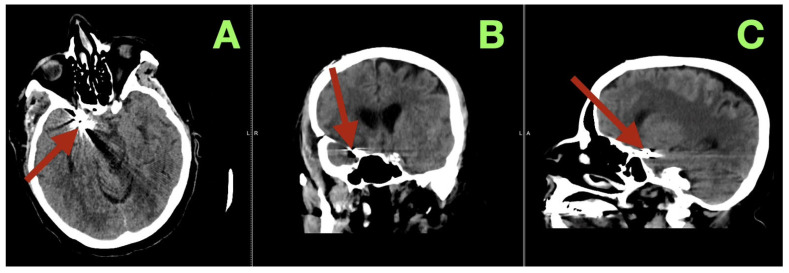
Postoperative day 5 non-contrast CT. (A) Axial section at the level of the skull base demonstrates the aneurysm clip at the right carotid–posterior communicating region (arrow), with expected localized metallic artifact and no evidence of recurrent subarachnoid hemorrhage, new intraparenchymal bleeding, or territorial hypodensity. The surrounding basal cisterns at this level remain discernible, without mass effect. (B) Coronal reconstruction confirms stable clip position within the right parasellar and suprasellar corridor (arrow), with preserved ventricular configuration and no radiographic signs of acute hydrocephalus or midline shift. (C) Sagittal reconstruction localizes the clip construct along the intended carotid–posterior communicating junctional plane (arrow), demonstrating anatomical coherence of the skull-base region and absence of secondary compressive changes.
